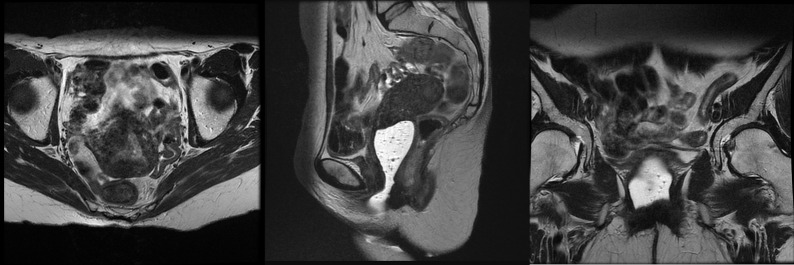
MR imaging following neoadjuvant chemoradiotherapy showing a near complete response. Axial, sagittal, and coronal images taken at the completion of concurrent chemoradiotherapy, prior to high-dose-rate brachytherapy. A 0.8 x 0.7 cm nodule was noted on the right side of the endometrial cavity, representing residual disease. All previously noted pelvic and retroperitoneal lymphadenopathy had resolved.
